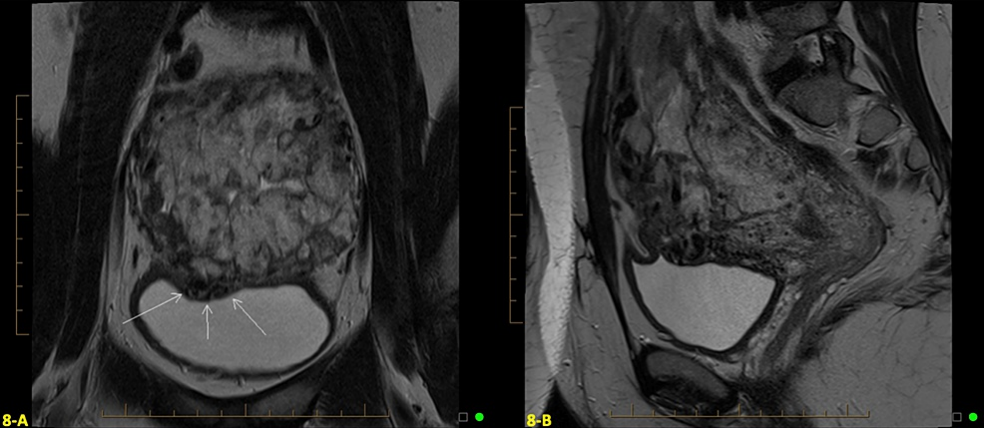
Axial (A) and Sagittal (B) T2 weighted magnetic resonance imaging (MRI) findings at four weeks postpartum A and B: Follow-up magnetic resonance imaging (MRI) at four weeks postpartum revealed a persistent placenta percreta to the anterior dome of the bladder demonstrated by the arrows.
